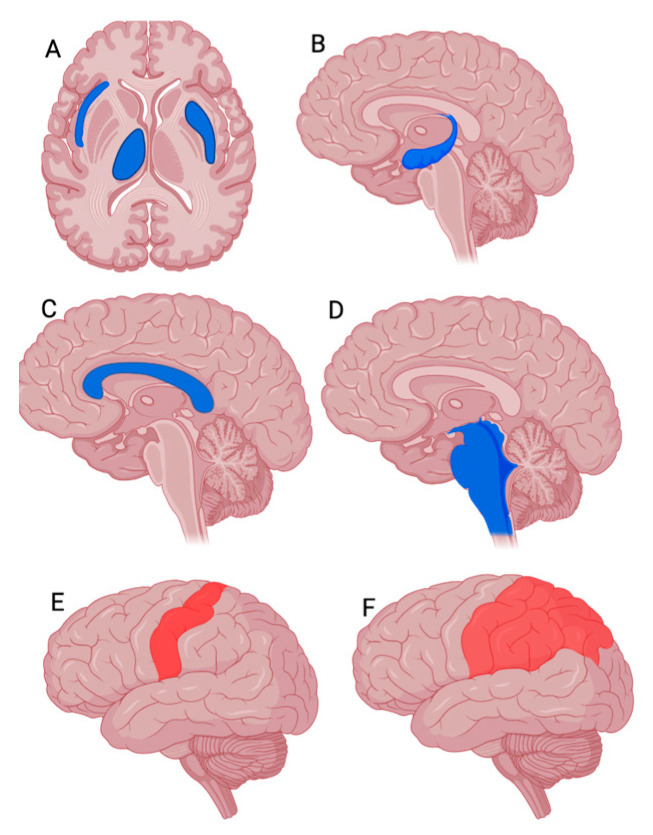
Representative PET findings in FMS patients compared to healthy controls. Created using BioRender.com. (A,B) [18F]FDG PET images (axial and sagittal slices): regions in blue indicate reduced glucose metabolism in the right thalamus, right insula, and left striatum (axial), and hippocampus (sagittal) in FMS patients. (C,D) [18F]DOPAPET images (sagittal slices): blue regions represent decreased presynaptic dopaminergic activity in the limbic system and brainstem. (E,F) TSPO PET images (e.g., [11C]PBR28 or [18F]DPA-714; sagittal slices): red regions indicate increased tracer uptake reflecting neuroinflammation in the primary somatosensory cortex and right parietal gray matter. Color scale interpretation: blue areas indicate reduced tracer uptake in FMS patients compared to healthy controls; red areas indicate increased uptake.
